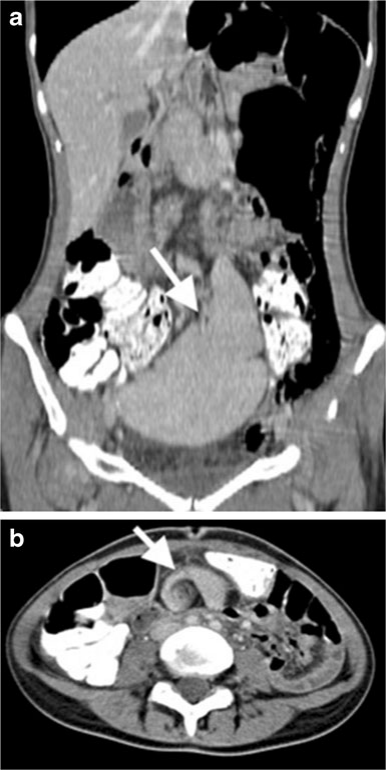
Splenic torsion in a patient with wandering spleen. (a) Coronal contrast-enhanced CT image reveals ‘ectopic wandering spleen’ (*arrow*) in the pelvis. (b) Axial contrast-enhanced CT at superior level than pelvis further demonstrates *‘whirling’* appearance (*arrow*) of splenic vessels, suggesting *‘torsion of the spleen’* as well. Normal parenchymal enhancement indicates lack of infarction
